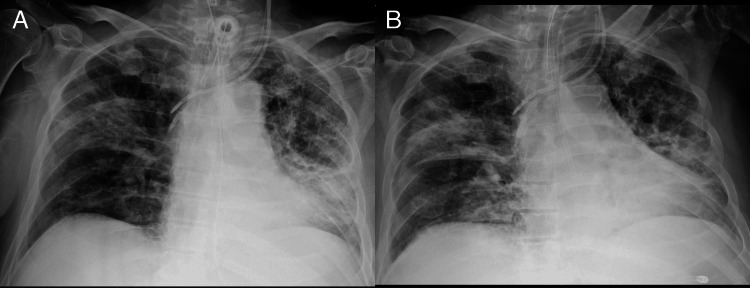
Serial chest radiographs showing late-stage recovery after VV-ECMO decannulation in ARDS due to TB A chest X-ray taken on day 21, 10 days after VV-ECMO decannulation, showed further resolution of bilateral lung opacities and ongoing lung recovery (A). On day 28, just before discharge, imaging revealed little further resolution, with residual lung opacities still present (B). VV-ECMO: venovenous extracorporeal membrane oxygenation, ARDS: acute respiratory distress syndrome, TB: tuberculosis
